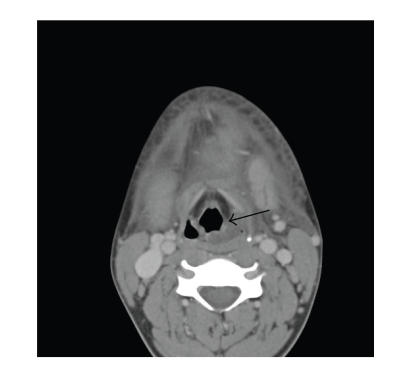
CT-scan demonstrating fluid collections in the left lateral pharyngeal
